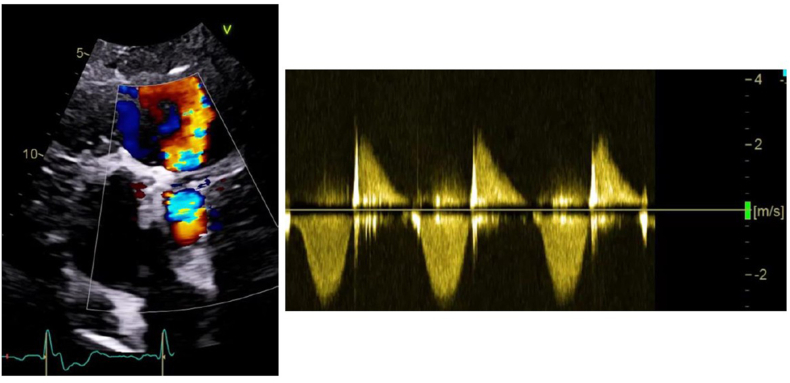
Color doppler and continuous doppler showing a severe pulmonary regurgitation in a Melody™ valve (in Contegra™) in a multioperated patient wit tetralogy of fallot.
